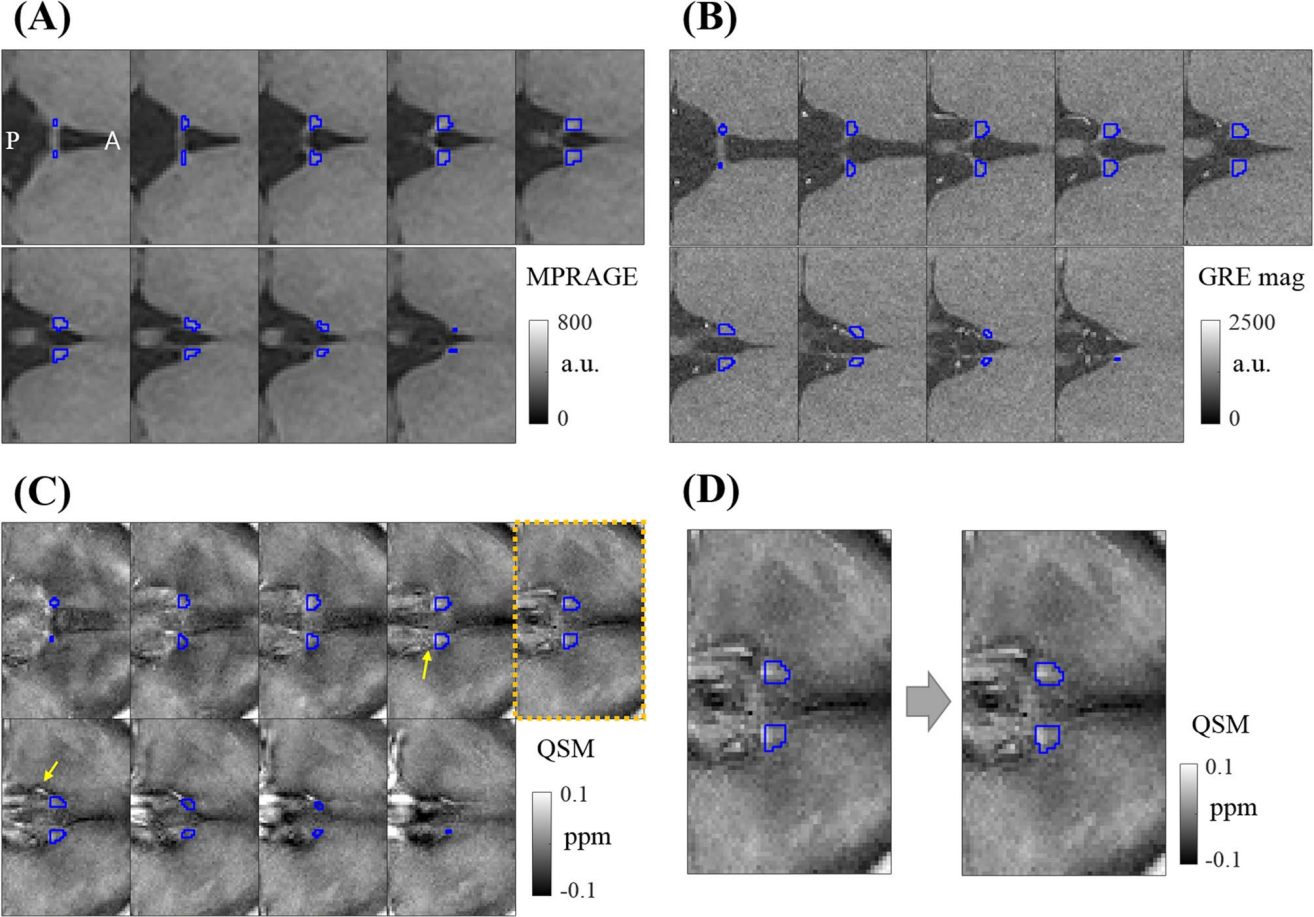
(A) Habenula ROI on T1-weighted MPRAGE images following the Lawson’s method. All images are shown on the axial plane, with the anterior side to the right. (B) Habenula ROI registered onto GRE magnitude images. (C) Same ROI on QSM. Suspected vein-like structures are marked with yellow arrows. The highlighted slice (rightmost) indicates the slice chosen for further analysis (minimum-vein slice). (D) Posterior edge adjustment of the habenula ROI on the chosen slice.
